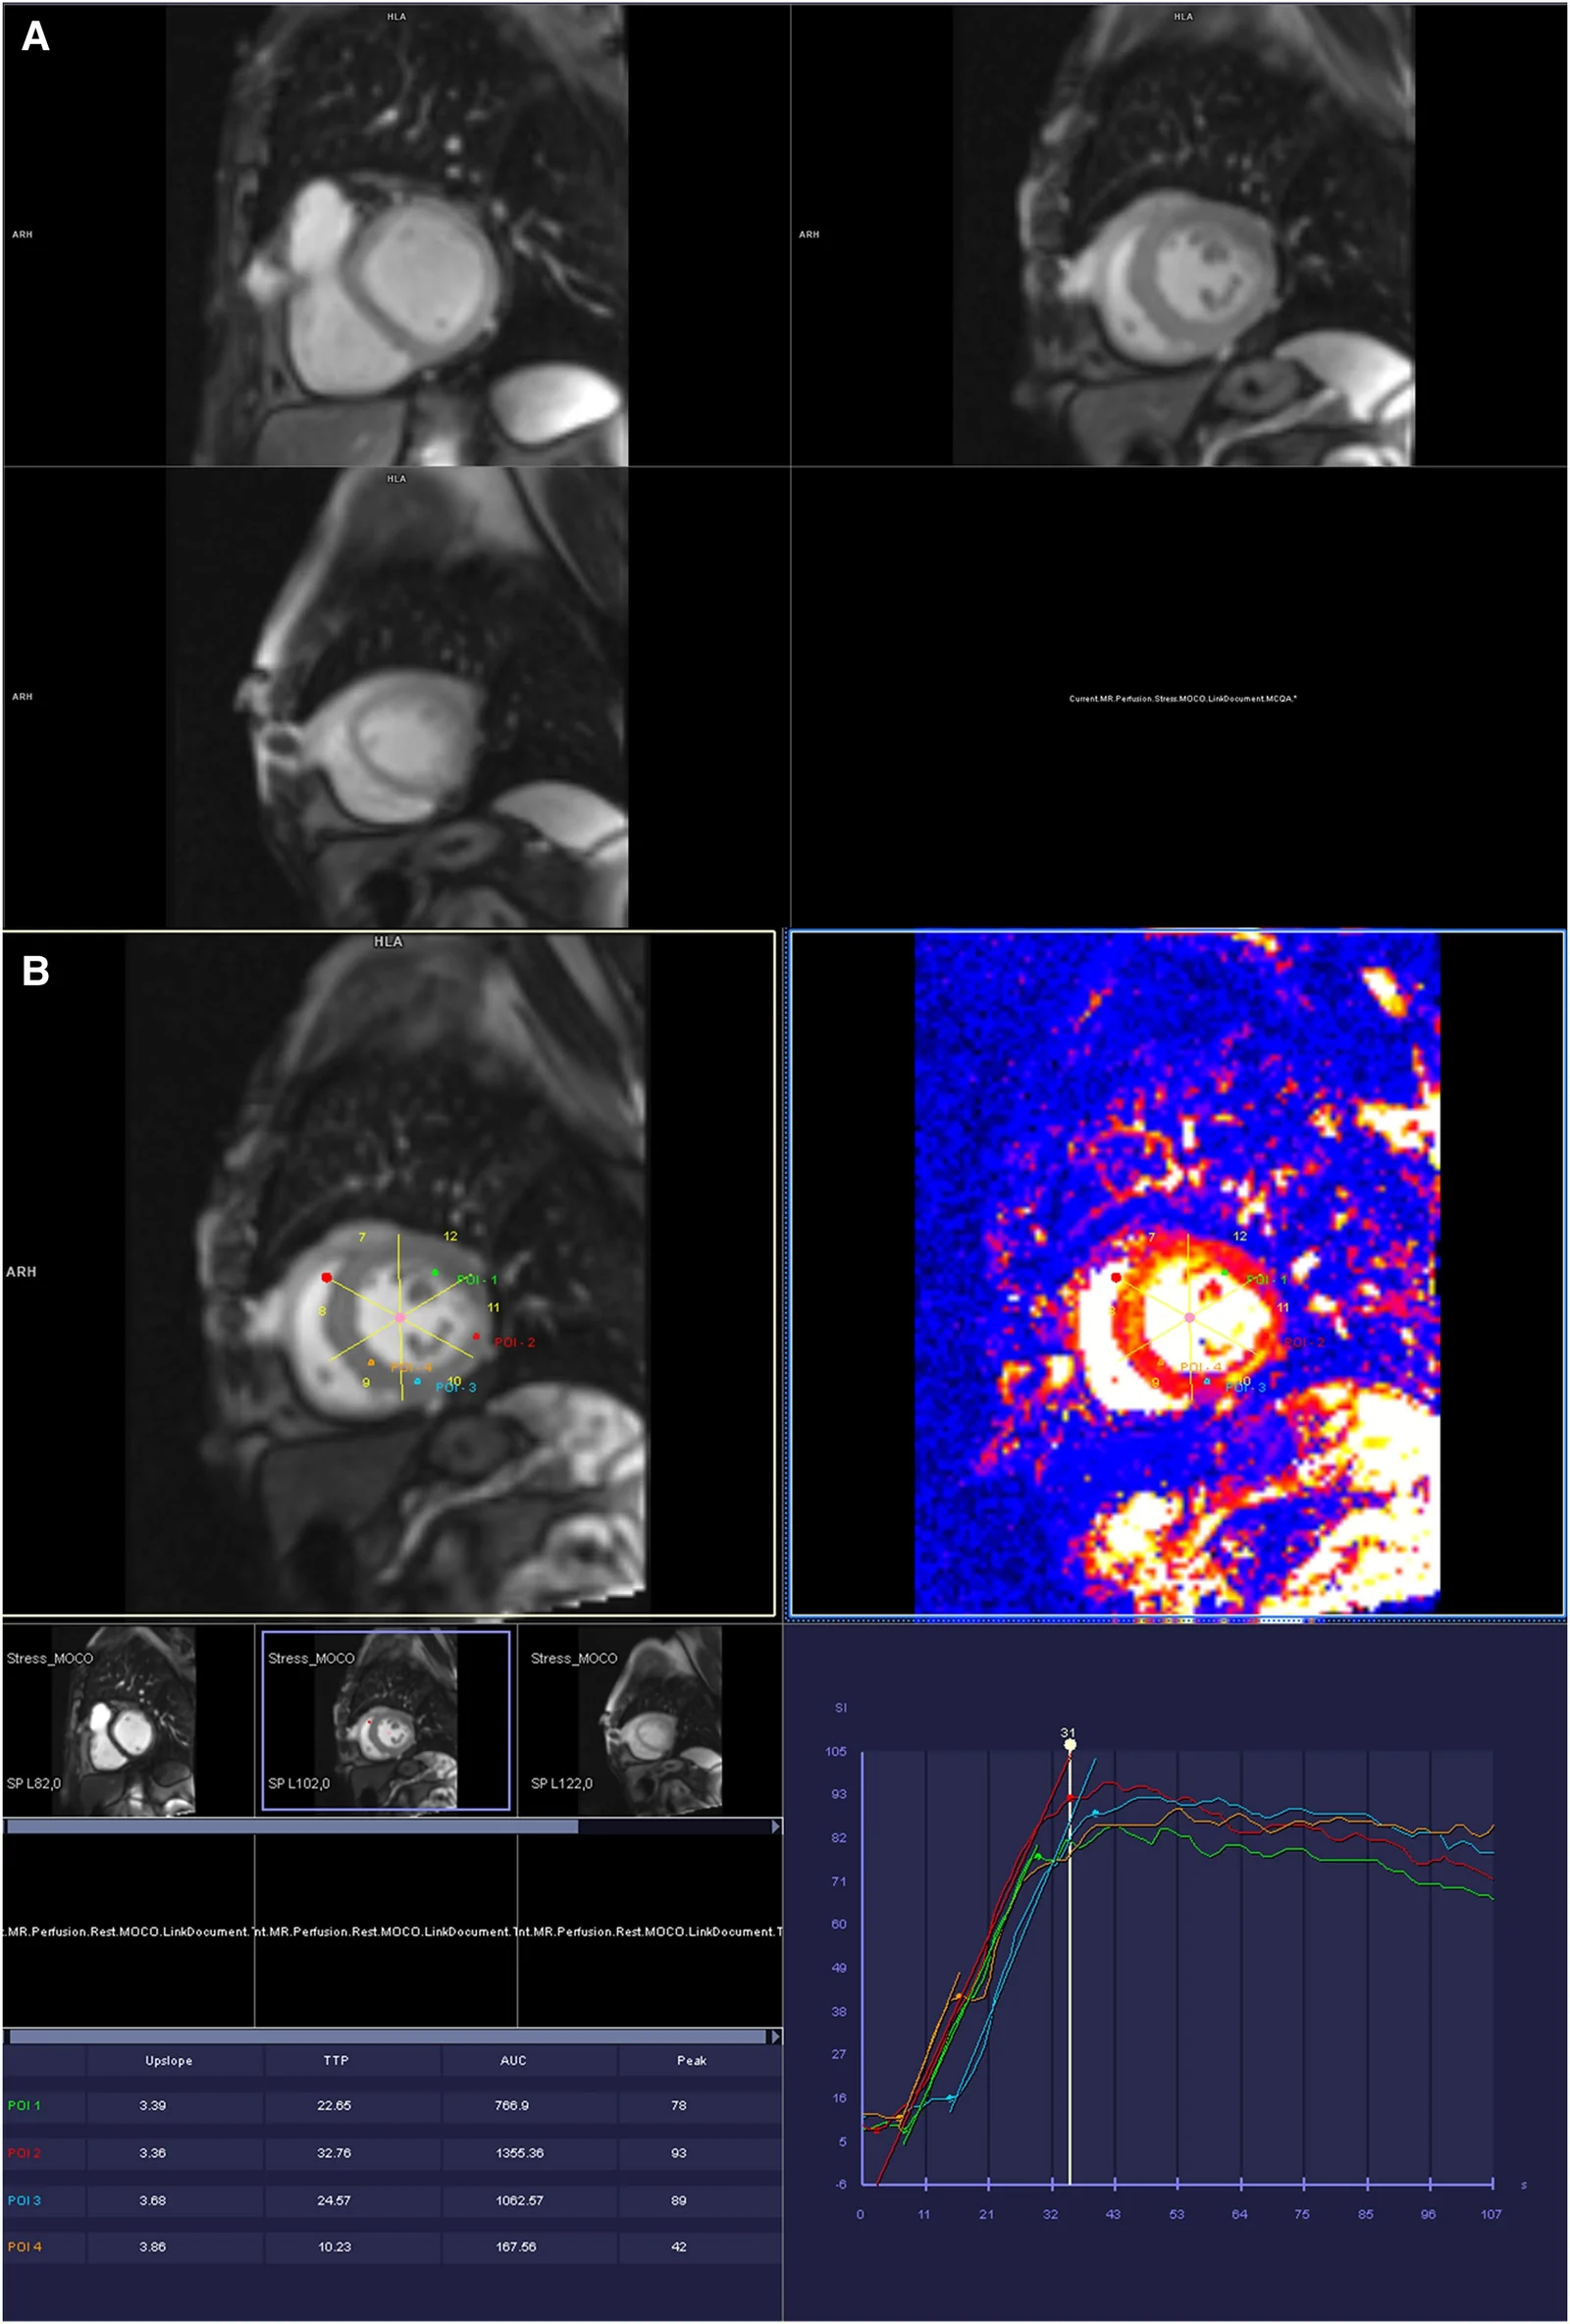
Adenosine stress perfusion cardiac magnetic resonance imaging. (*A*) Qualitative assessment of stress perfusion imaging in short-axis view showing homogeneous myocardial enhancement without regional perfusion defects. (*B*) Semi-quantitative perfusion analysis demonstrating normal myocardial perfusion curves without evidence of inducible ischaemia.
